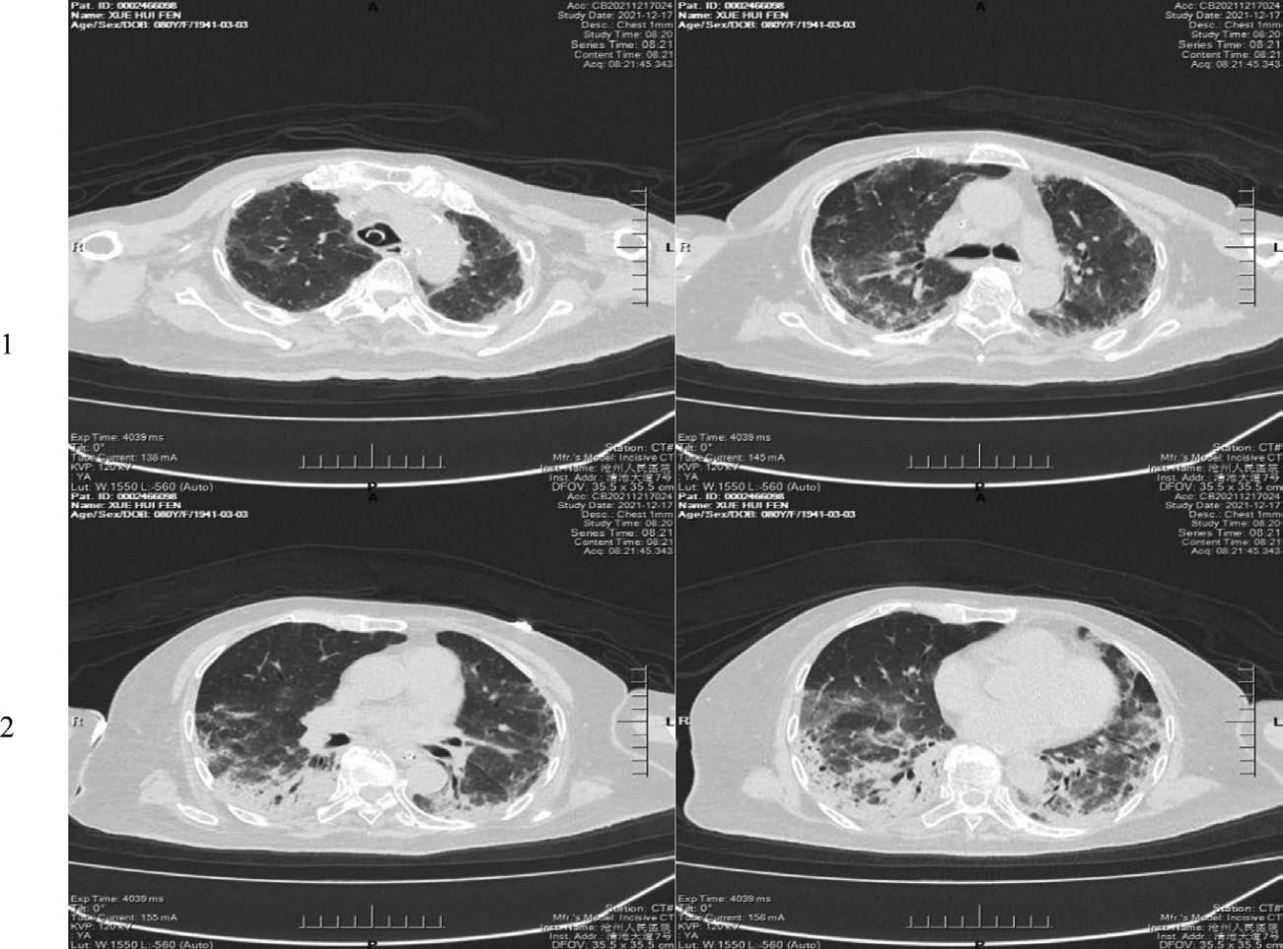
Results of CT scans of the lungs after anti-infective treatment showed that the inflammatory exudation and solid lesions almost completely disappeared. CT = computed tomography.
